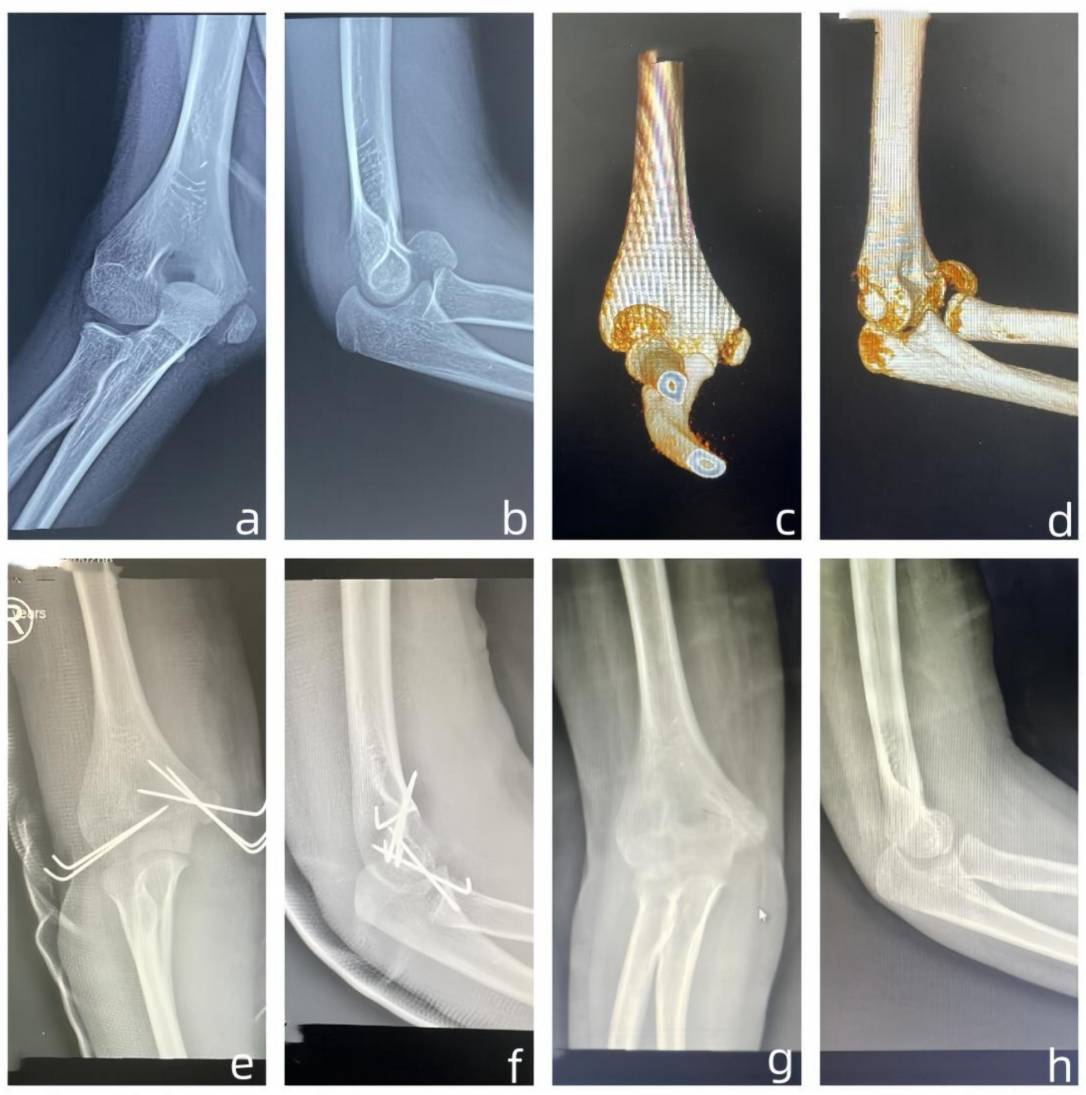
(a) One 13 years girl, traffic accidents, 1 day after injury the Anteroposterior radiographs; (b) 1 day after injury the Lateral radiographs; (c) 1 day after injury the Anteroposterior Three dimensional CT; (d) 1 day after injury the Lateral Three dimensional CT; (e) 1 day post operation the Anteroposterior radiographs; (f) 1 day post operation the Lateral radiographs; (g) 4 months post operation the Anteroposterior radiographs; (h) 4 months post operation the Lateral radiographs.
